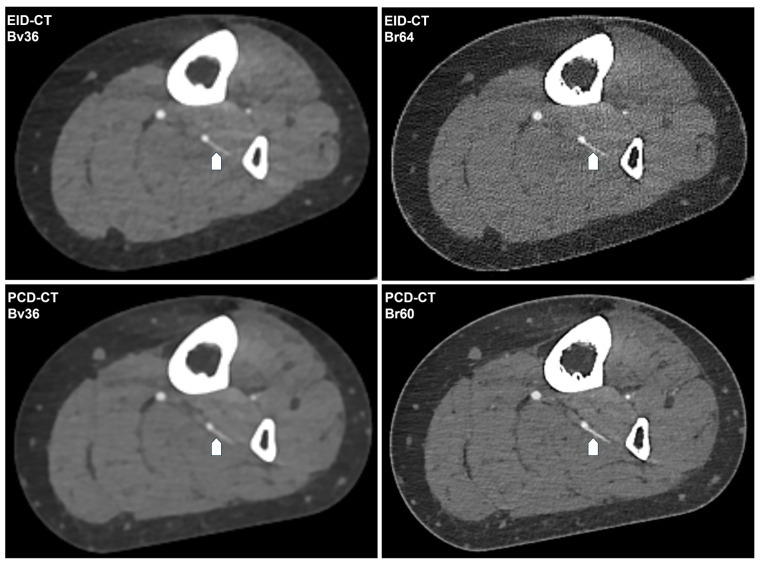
Intraindividual comparison of the CT angiography image quality. The following CT angiography images of a 62-year-old female patient’s lower limb are generated using EID-CT or PCD-CT and reconstructed with the investigated convolution kernels. The same fibular perforator artery is indicated by a white arrow. Window setting: window width 700 HU; window center 100 HU. Bv, body vascular kernel; Br, body regular kernel; EID-CT, energy-integrating CT; HU, Hounsfield units; PCD-CT, photon-counting CT.
